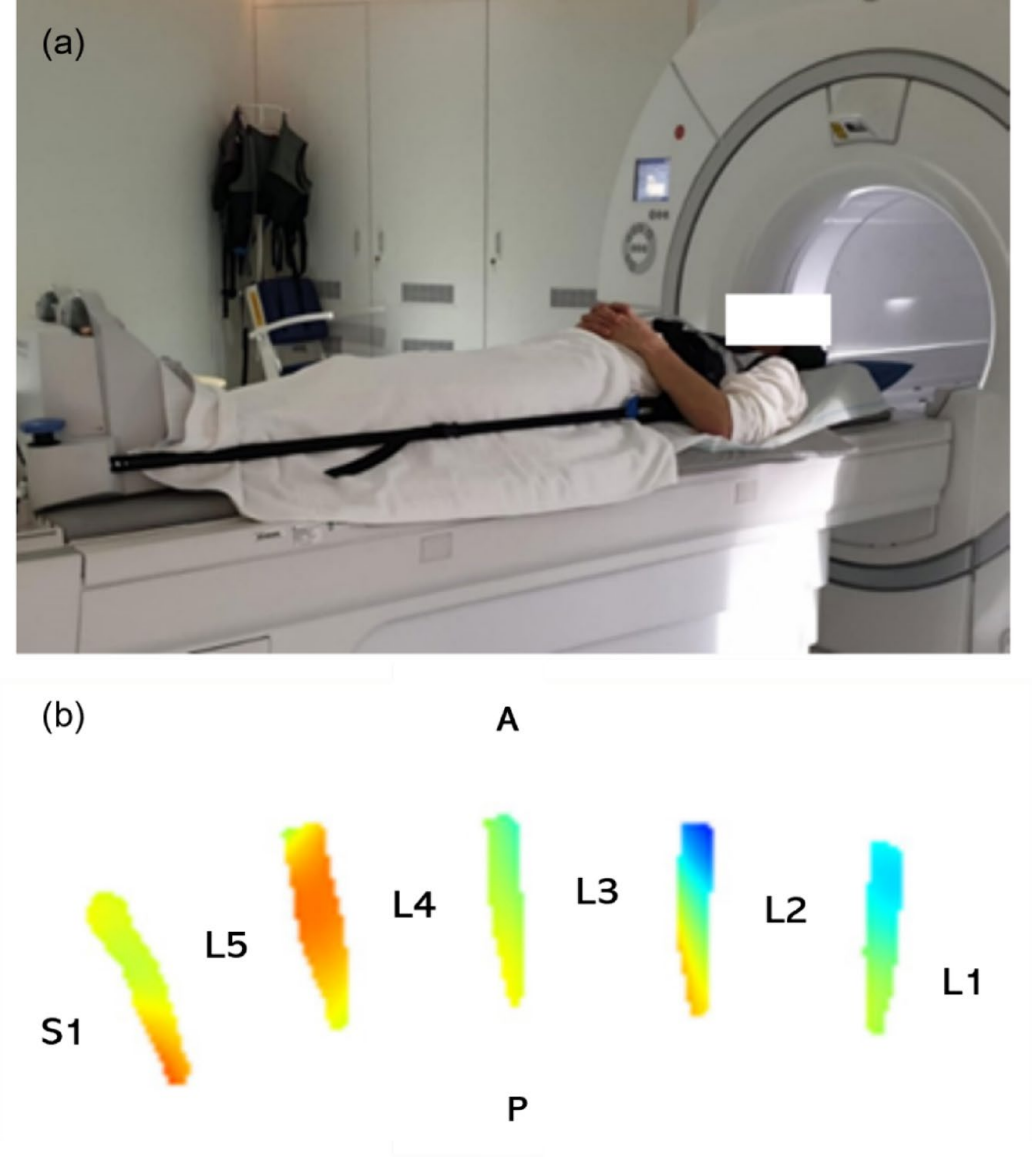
(a) A participant lies in the supine position on the tabletop of a whole-body MRI scanner with the Dynawell Diagnostics^®^ compression device applied to axially load the spine during the examination. (b) The deformation of the intervertebral discs during the spinal loading can be quantified by registering MR images with and without spinal loading to calculate the local compression (red) and expansion (blue) in terms of the Jacobian determinant of the registration deformation field. Written informed consent has been obtained from the participant for permission to use the actual image.
